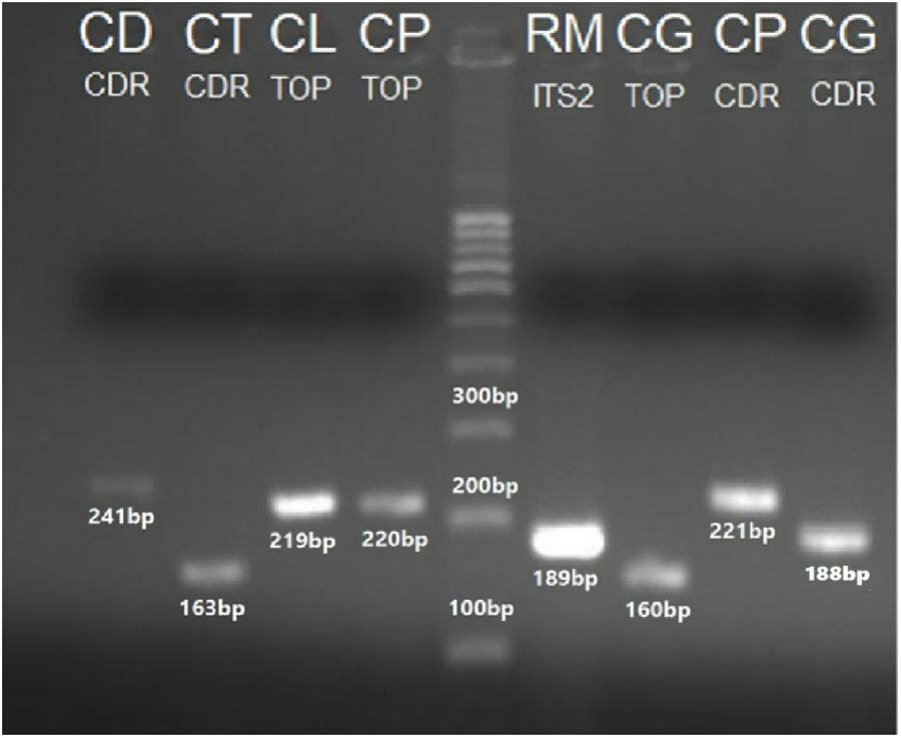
PCR amplified products through specie-specific primers of Topoisomerase II gene: CL(TOP) = *C*. *lusitaniae* (219 bp), CP(TOP) = *C*. *parapsilosis* (220 bp), CG(TOP) = *C*. *glabrata* (160 bp); CDR gene: CD(CDR)= *C*. *dubliniensis* (241 bp), CT(CDR)= *C*. *tropicalis* (163 bp), CP(CDR)= *C*. *parapsilosis* (221 bp), CG(CDR)= *C*. *glabrata* (188 bp); and D=100 bp ladder.
